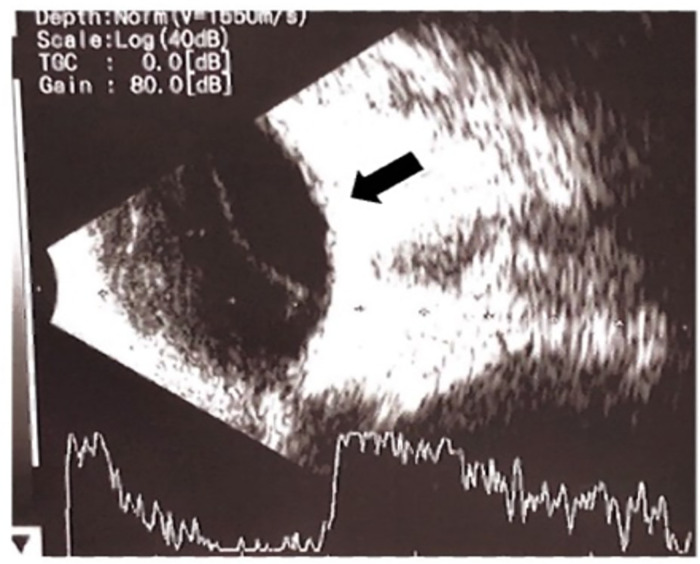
Ultrasound examination of the left eye showed pan-uveitis developed exudative retinal detachment.
